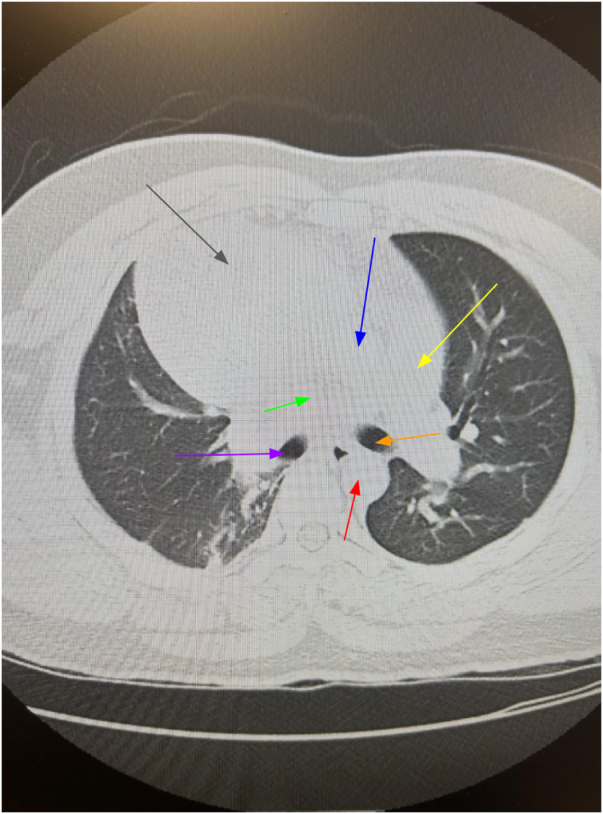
Axial CT scan of the chest (lung window) demonstrating a well-circumscribed, heterogeneous anterior mediastinal mass measuring approximately 7.5 cm × 5.2 cm × 6.8 cm. Gray arrow: mediastinal mass; Green arrow: superior vena cava compression; Blue arrow: ascending aorta; Red arrow: descending aorta; Yellow arrow: pulmonary artery trunk; Purple arrow: right mainstem bronchus; Orange arrow: left mainstem bronchus. The lungs show no focal airspace infiltrates, pleural effusion, or pneumothorax. No osseous or chest wall abnormality. The mass abuts but does not invade adjacent vessels or the heart, consistent with mediastinal lymphangioma. CT, computed tomography.
